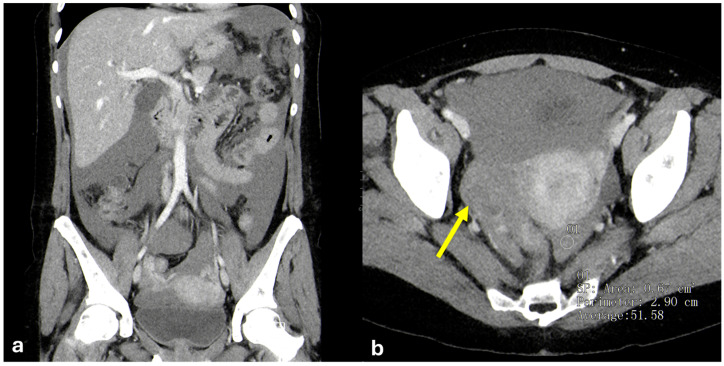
Hemoperitoneum. A young woman with acute subacute abdominal pain, anemisation, and no history of trauma. Free fluid was detected at ultrasound and portal venous phase abdominal CT was requested. Coronal CT reconstruction (a) shows a diffuse peritoneal effusion. On axial reconstruction (b), the peritoneal fluid shows high attenuation values in the dependent portions (52 HU). Moreover, the right ovary appears increased in size (arrow). The patient underwent explorative laparotomy, and a ruptured luteal cyst in the right adnexa was detected as the cause of the hemoperitoneum.
